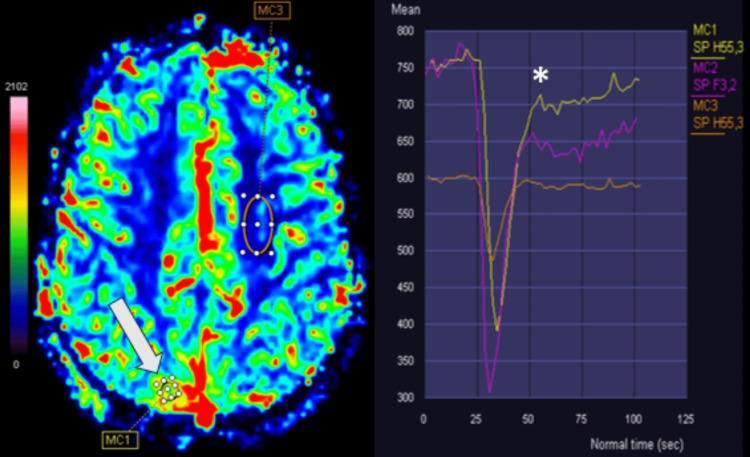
Dynamic perfusion MRI study of the lesion in the right posterior parasagittal parietal convexity (white arrow) shows lower-than-expected rCBV (3.6) and intensity time curve with partial return to baseline (asterisk) with a percentage signal recovery of 86%. All these features were not compatible with the predictable values for a meningioma MRI: magnetic resonance imaging; rCBV: relative cerebral blood volume
